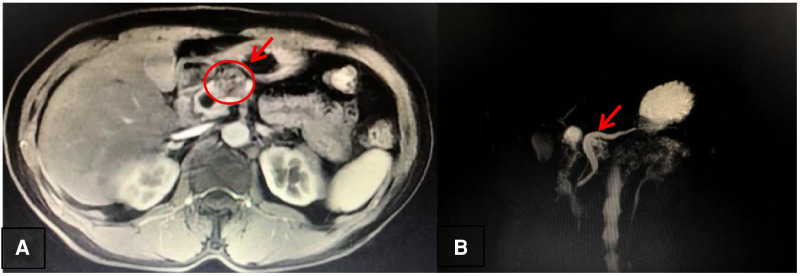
A: Magnetic resonance imaging. B: Magnetic resonance cholangiopancreatography..
